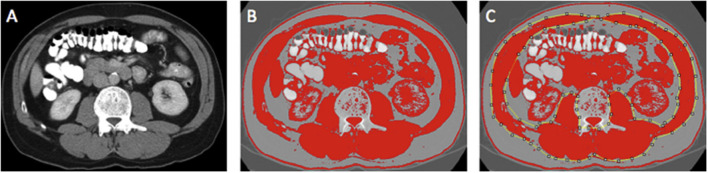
Example of selection of CT body composition—skeletal muscle area (SMA) using ImageJ software; (A) mid-L3 vertebra axial slice from preoperative portal venous phase CT; (B) threshold selection of skeletal muscle tissue using automatic selection of pixels of radiodensity ranging _29–150 Hounsfield units (HU); (C) region of interest (ROI) selection for SMA (cm^2^). Adapted from McSorley et al. (22).
